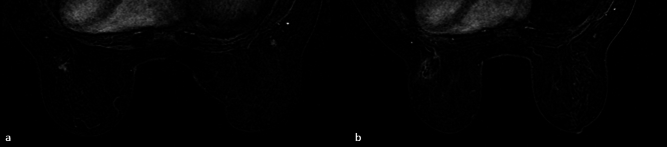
Axial T1-weighted post-contrast subtracted MR image shows a 12 mm invasive breast carcinoma in the left breast before cryoablation; b the cryoablated area after 11 days
